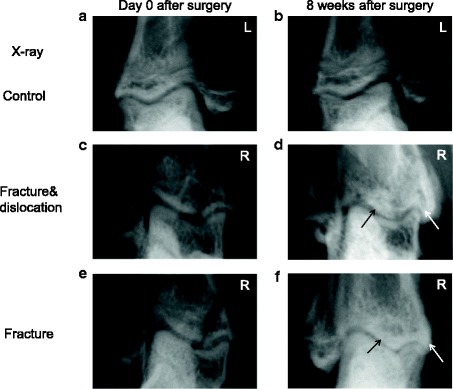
Radiography demonstrated the OA changes in the ankle joint 8 weeks after surgery (b, d, and f) when compared with immediately after surgery (a, c, and e). d and f suggested that the joint space became narrow. Black arrow shows subchondral sclerosis and white arrow shows osteophytes
